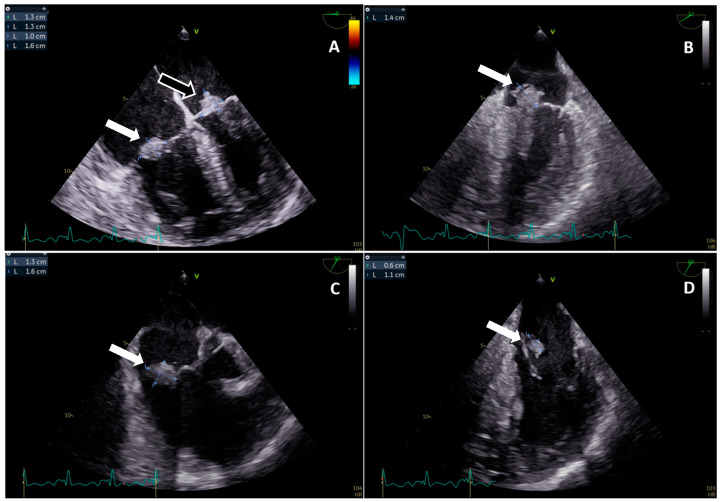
(A–D). Transoesophageal echocardiography performed in our hospital. (A): Mid-esophageal four-chamber view at 0° in TOE showing a 16 × 10 mm isoechoic vegetation on tricuspid posterior leaflet (white arrow) and another 13 × 13 mm isoechoic vegetation on mitral posterior leaflet (black arrow). (B): Mid-esophageal off axis view at 30° in TOE showing an isoechoic 14 mm vegetation on posterior mitral leaflet. (C,D): Mid-esophageal mitral commissural view at 60° in TOE showing an isoechoic vegetation on atrial side of mitral P3 scallop in different cardiac cycles (systole (C) and diastole (D)).
